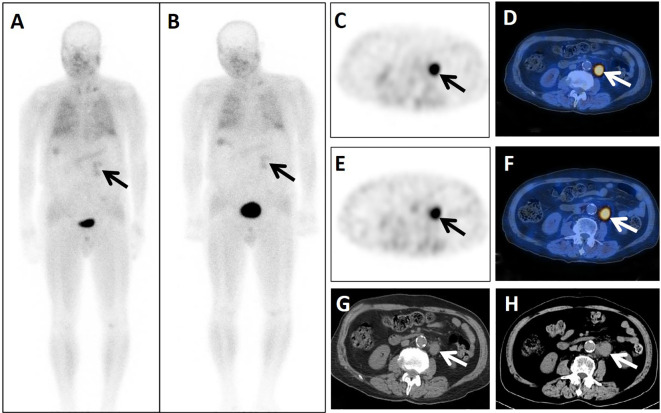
A 74-year-old male patient presented 4 years after surgery for rectal cancer. He underwent dual-phase ^99m^Tc-FAPI-04 SPECT/CT. The early anterior whole-body MIP image (A, arrow) showed abnormal accumulation of radioactivity in the left upper abdomen. The corresponding axial SPECT image (C, arrow) and fused ^99m^Tc-FAPI-04 SPECT/CT image (D, arrow) of the lesion showed high uptake of ^99m^Tc-FAPI-04 with TBR = 7.06. The lymph node was shown more clearly in the delayed anterior whole-body MIP image (B, arrow), corresponding axial SPECT image (E, arrow), and fused ^99m^Tc-FAPI-04 SPECT/CT image (F, arrow). The lesion showed higher uptake of ^99m^Tc-FAPI-04 with TBR = 7.68. The axial CT image (G, arrow) showed a lymph node measuring 32 mm × 22 mm in the left beside of abdominal aorta. Lymph-node metastasis was confirmed by follow-up CT (H, arrow) 4 months later, measuring 39 mm × 26 mm with increased levels of the tumor markers CEA (18.69 ng/mL), CA724 (27.77 IU/mL), and CA199 (48.89 U/mL).
